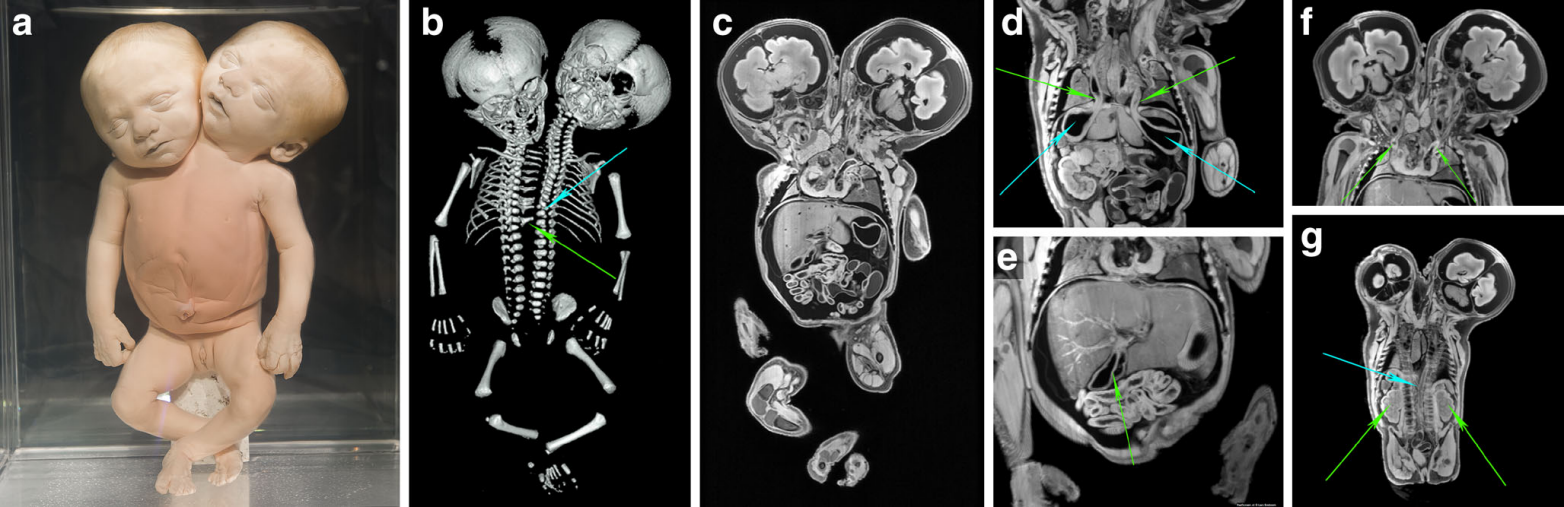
a Photograph of the full-term conjoined twin of case 3. b Three-dimensional reconstructed skeleton based on the CT data showed butterfly and block vertebra (*turquoise arrow*) with fused ribs (*green arrow*) between the two separate vertebral columns There were two heads, two arms and two legs with one broad pelvis. c Coronal T1-weighted MR image showed two normal brains, one shared “fused” heart and liver with one overarching diaphragm. d Coronal T1-weighted MR image showed two separate oesophagi (*green arrows*) and two separate stomachs (*turquoise arrow*). e Coronal T1-weighted MR image showed a “fused” liver with two gallbladders (*green arrow*). f Coronal T1-weighted MR image showed two ascending aortas (*green arrows*). g Coronal T1-weighted MR image showed two kidneys and adrenal glands (*green arrows*) and one anus. The two descending aortas fused at the level of the 11th thoracic vertebra (*turquoise arrow*). Note that the right descending aorta is smaller than the left descending aorta
